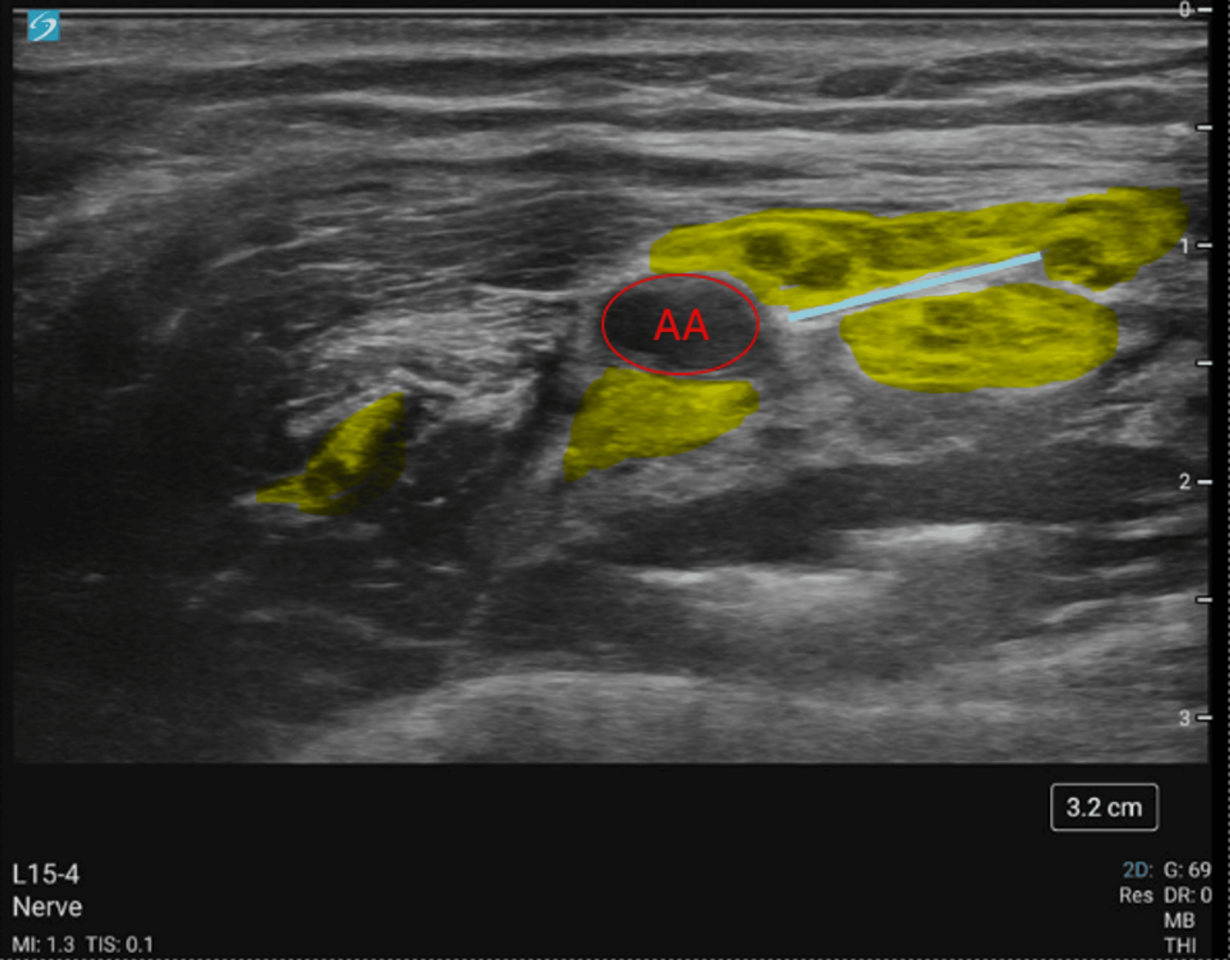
Labeled ultrasound-guided axillary nerve block on the patient with a 15-4 MHz linear transducer and a 20-gauge, 4-inch echogenic B. Braun Ultraplex 360® needle. The axillary vein was compressed in the ultrasound snapshot The red circle highlights the axillary artery (AA). Yellow areas highlight the brachial plexus. The blue rectangle highlights the collapsed axillary vein.
